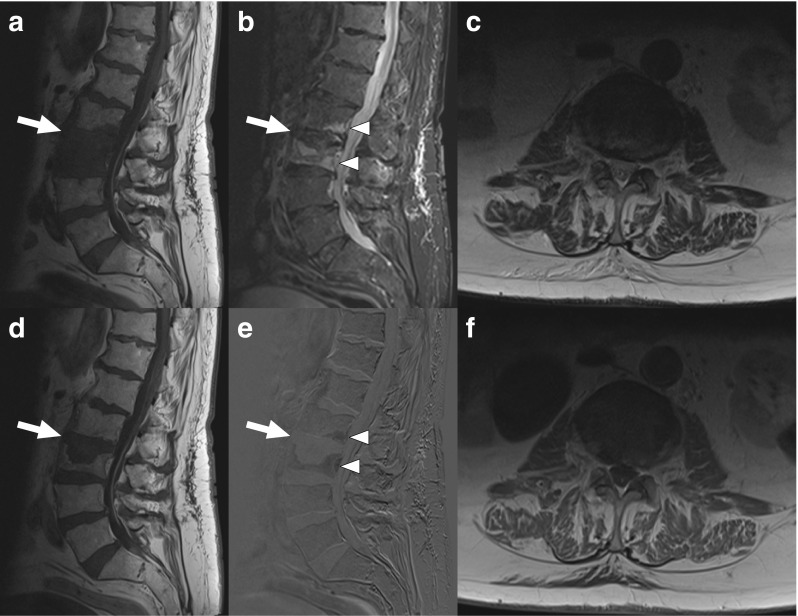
A 88-year-old man with MRI findings atypical of spondylodiscitis. Sagittal T1-weighted (a), fat-suppressed T2-weighted (b), gadolinium-enhanced T1-weighted (d), and gadolinium-enhanced subtraction images (e) show pathological signals in both the L2 and L3 vertebrae (*arrows* and *arrowheads*), but no clear T2 hyperintensity or gadolinium enhancement of the L2–L3 disc. Axial T2-weighted (c) and gadolinium-enhanced T1-weighted images (f) do not show any paravertebral phlegmon or abscess either. CT-guided biopsy was culture-negative
